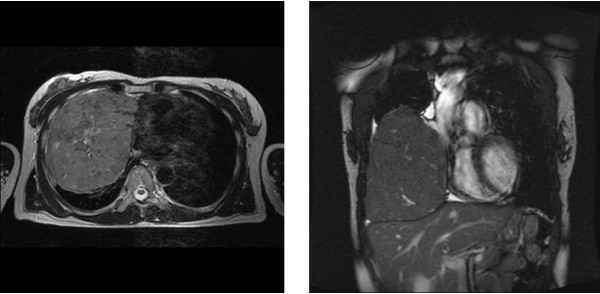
Chest magnetic resonance imaging. The tumor appeared isointense relative to the skeletal muscle on T1-weighted image, while T2-weighted images show partial inclusion of weak signal hypointensities of moderate signal strength.
